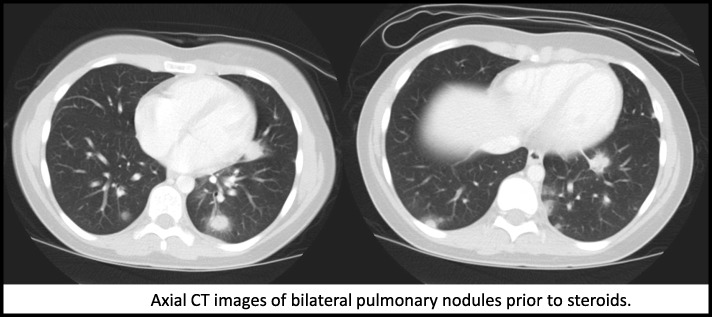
Axial CT images of bilateral pulmonary nodules prior to steroids
